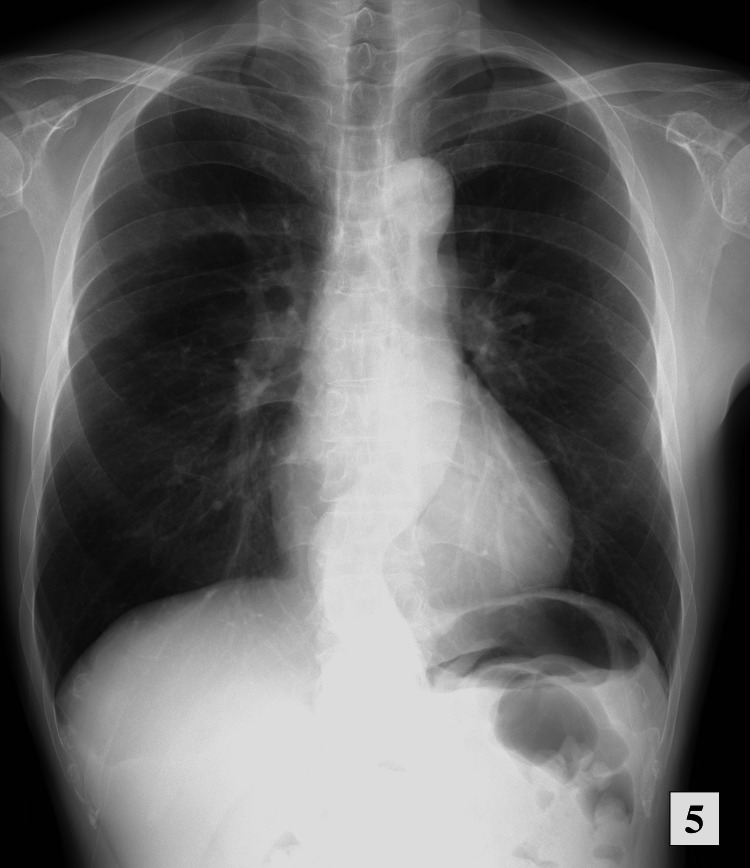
Chest radiograph five months after discharge showing significant improvement and no recurrence.
